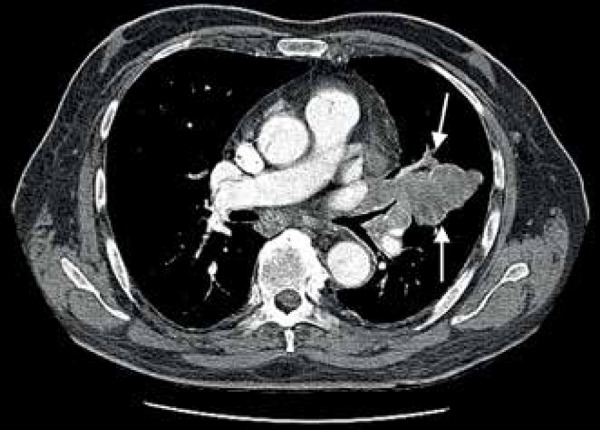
Postoperative thoracic CT. Metastatic tumor (arrows) located in the left
